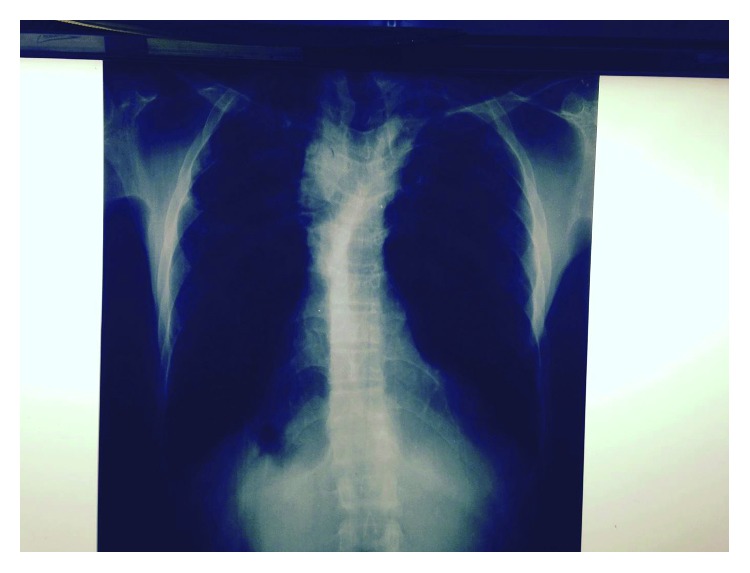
Chest X-ray after 17 months showing a fully expanded lower lobe and the same fibrosis in both lungs with no change.
